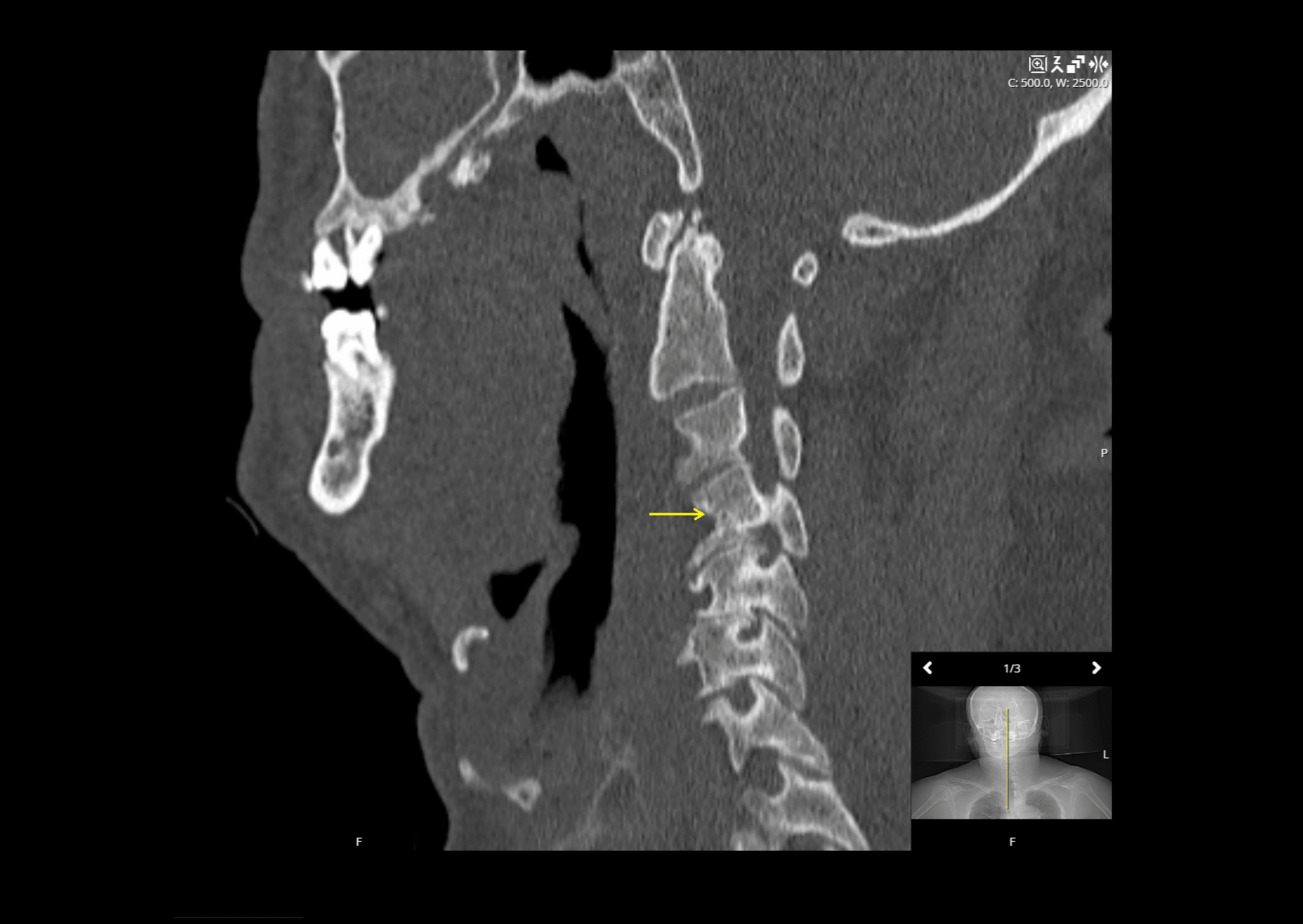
Sagittal CT of the cervical spine The preliminary report suggested a mildly displaced acute fracture involving the anterior inferior endplate of C4 (indicated by the yellow arrow). Subsequent formal review amended this to show chronic spondylotic changes without evidence of acute bony disruption CT: computed tomography
